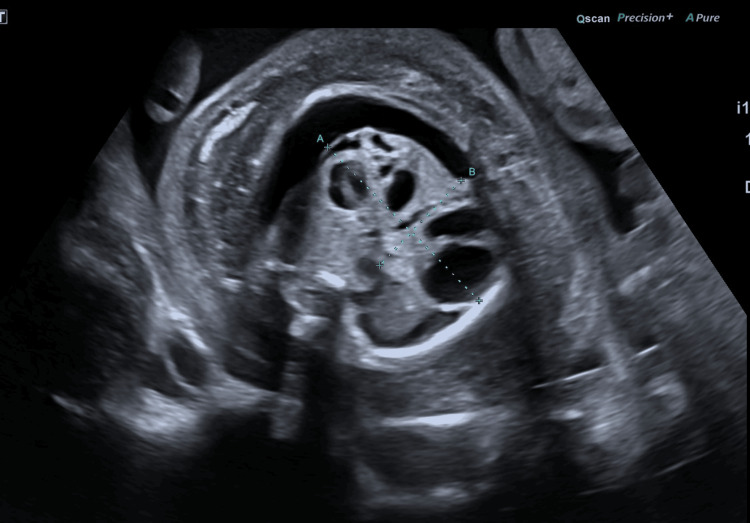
Transverse ultrasound view of the thorax demonstrating an irregular, multiseptated mass with a minor solid component, measuring 38.5 x 21 mm.
